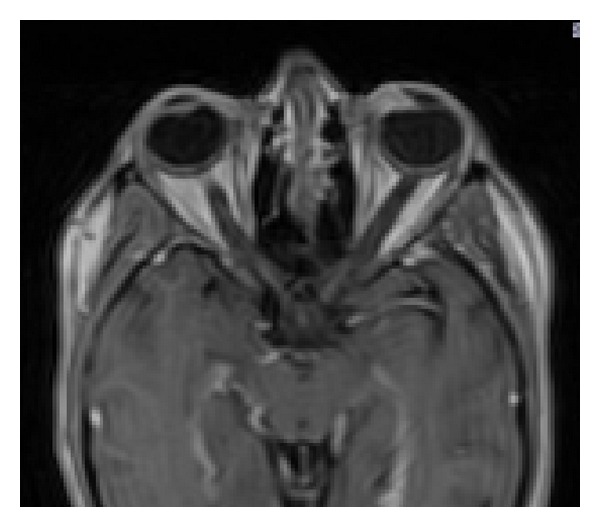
Magnetic resonance imaging scan demonstrates an anterior scleral mass behind the iris plane and with nasal scleral bulge.
